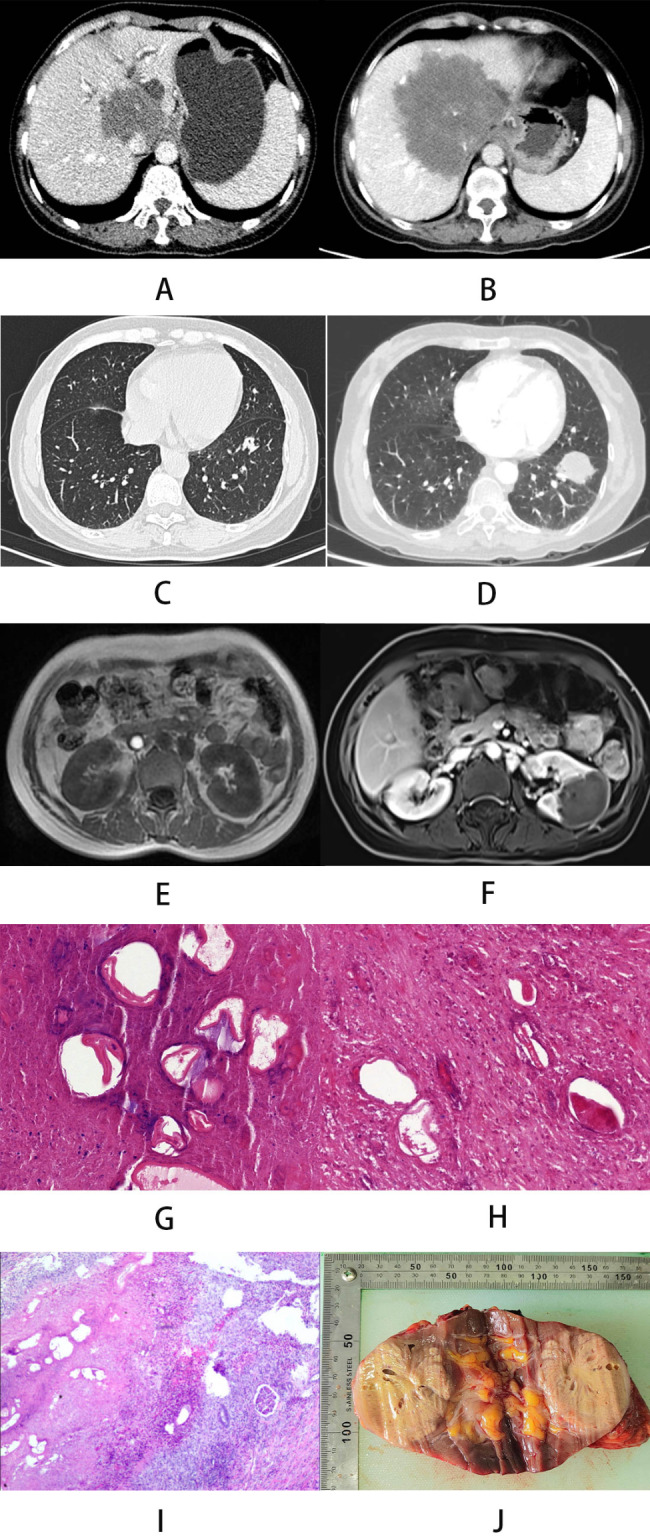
CT and MRI manifestations of the lesion and pathological examination and macroscopic view of the excised: (A) CT image of hepatic alveolar echinococcosis in 2017; (B) 2020 Liver lesions were more invasive than before; (C) CT images of lung lesions in 2017; (D) Enlargement of the left lung lesion in 2020; (E) MRI images of the kidneys did not show abnormalities in 2020; (F) MRI images suggestive of renal space-occupying lesions in 2020; (G) Pathologic examination of liver lesion location; (H) Pathologic examination of lung lesion location; (I) Pathologic examination of renal lesion location; (J) Macroscopic view of left renal lesion
